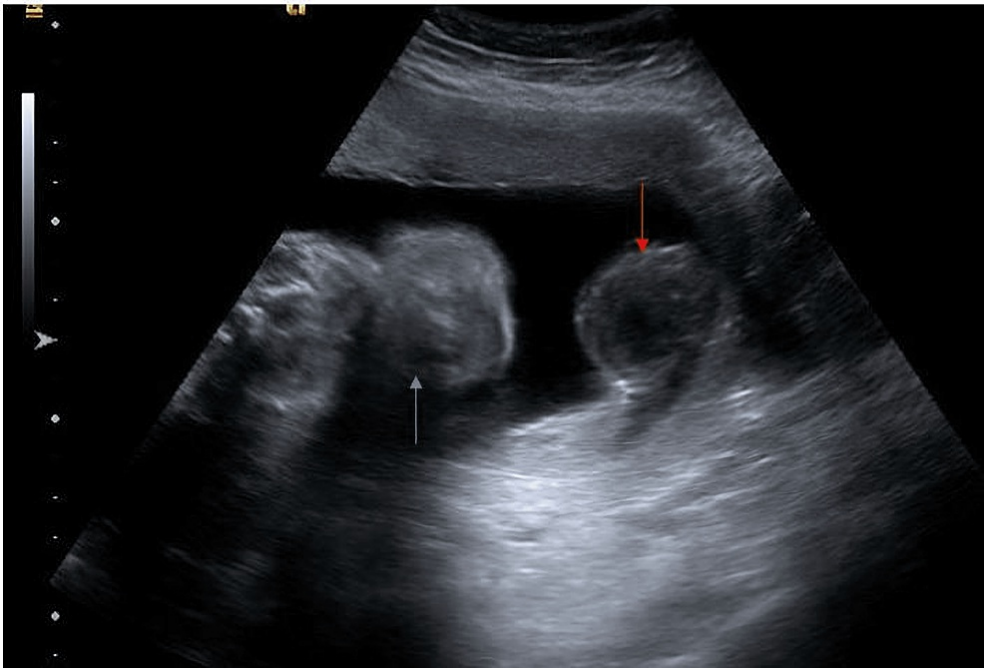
Ultrasound at 28 weeks The gray arrow shows the head of the fetus. The red arrow shows the left fallopian tube endometrioma
